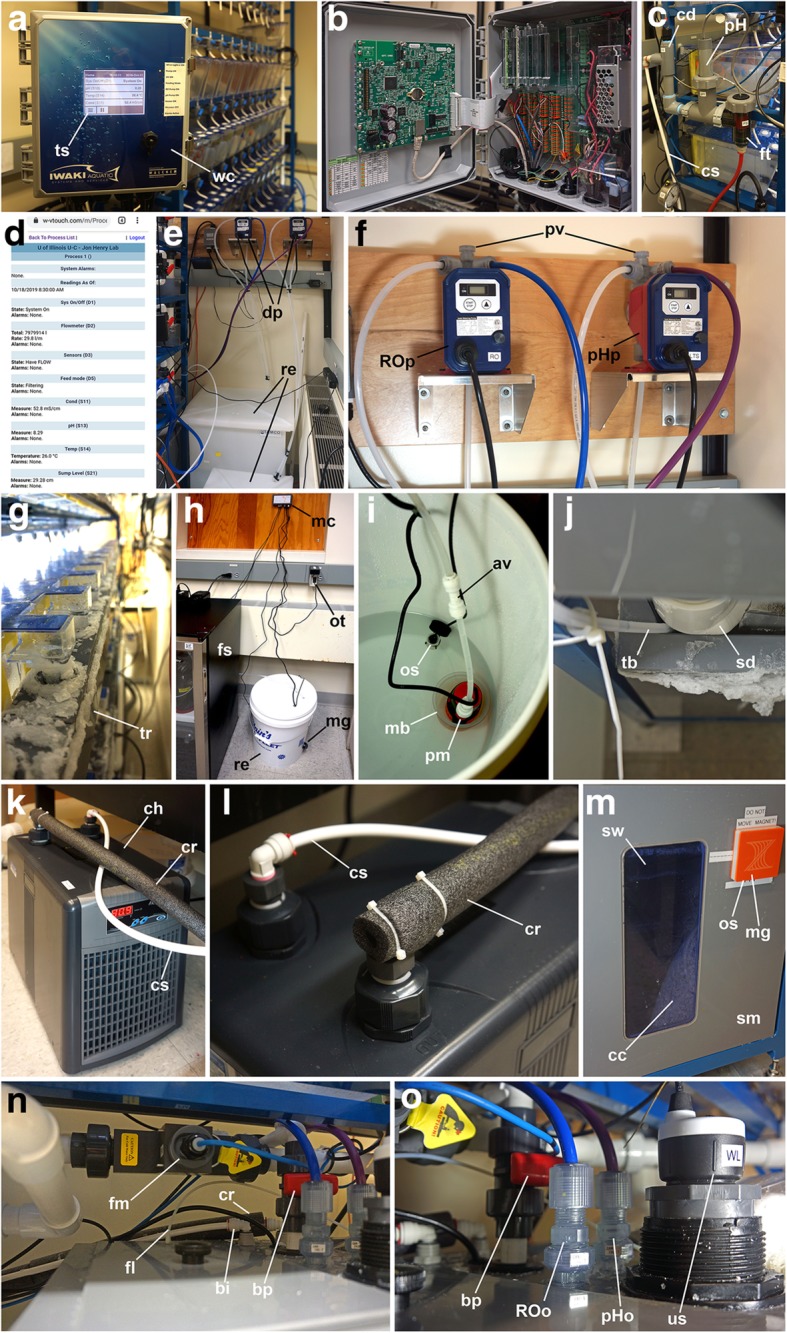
a–o Overview of the Walchem 900 controller, main sensors, dosing pumps, and the external chiller. a Walchem 900 controller and touch pad interface. b View inside the Walchem controller showing many electrical connections to the various sensors and dosing pumps, etc. c View of the sensor side loop. Water for the side loop is supplied by the red plastic tubing and passed to the external chiller by the white plastic tubing. d Example showing some of the information provided by the system, which can be viewed online. e View of the RODI water and pH/calcium dosing pumps and their reservoir, which are situated to the right of the rack system. f Close up view of the RODI water and pH/calcium dosing pumps, which are mounted on the wall. g Example of salt creep on one of the drain troughs. h View of the automatic seawater top off (ATO) unit. i View inside the seawater reservoir for the ATO unit showing the small submerged pump and optical water level sensor. j Location of the ATO unit supply line that directs seawater to the back right corner of the sump, just behind the slotted drain diverter. The tubing is secured with a pair of zip ties. Note evidence of salt creep. k View of the external chiller. Water for the chiller is supplied via the sensor side loop. The cold return line is covered with foam insulation. l Close-up view showing the custom fittings and right angle push connectors for the chiller’s water lines (see Additional file 3E). m View of the small window located in the sump, through which one can readily asses the water level. The Nylon mesh bags containing crushed coral can be seen inside the sump. The square magnet that secures the optical water level sensors for the ATO unit is also visible. The dual optical sensors are located inside the sump. n View of the flow meter and various connections to the sump. The food line is seen entering the sump through a small hole drilled in the PVC plastic lid that covers the left compartment. o View of the Echopod ultrasonic water level sensor and other connections to the sump. av., anti-siphon valve; bi, bioreactor return line; bp, bypass valve; cc, mesh bags containing crushed coral; cd, conductivity sensor; ch, chiller; cr, chiller return line; cs, chiller supply line; dp, dosing pumps; fl, feeding line; fm, flow meter; ft., float switch; mb, Nylon mesh basket; os, optical water level sensor; ot, outlet timer switch; pH, pH probe; pHo, pH dosing pump outlet; pHp, pH dosing pump; pm, ATO seawater dosing pump; pv priming valves; re, reservoir; ROo, RODI dosing pump outlet; ROp, RODI water dosing pump; sd, salt deposits; sw, sump window; SWo; seawater dosing pump outlet; tb, tubing from ATO unit; ts, touch screen; us, ultrasonic water level sensor. Other labels are the same as those used in Figs. 1 and 3
